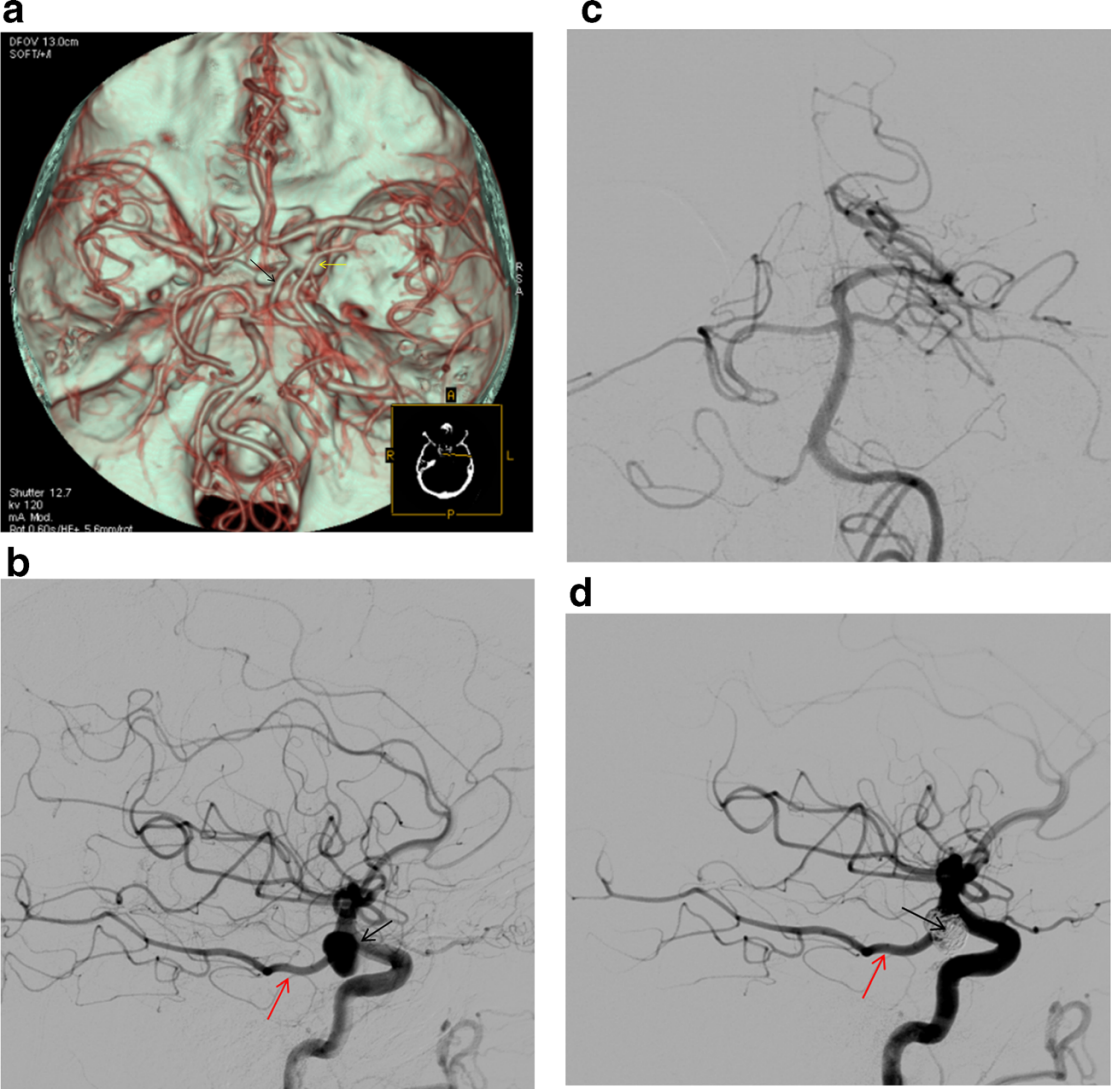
a CTA (VRT reconstructions) shows a right-sided foetal Pcom (*black arrow*) with a large aneurysm at the origin of the vessel (*yellow arrow*). b Digital subtraction angiography (*lateral view*) of the right ICA shows a foetal Pcom (*red arrow*) and large aneurysm at the origin of the vessel (*black arrow*), which cannot be compromised. c Digital subtraction angiography (*AP view*) of the left vertebral artery. The posterior cerebral artery on the right is not opacified (definition of a foetal Pcom). b Post-embolisation digital subtraction angiography (*lateral view*) of the right ICA shows good patency of the foetal Pcom (*red arrow*) and complete obliteration of the aneurysmal sac (*black arrow*)
